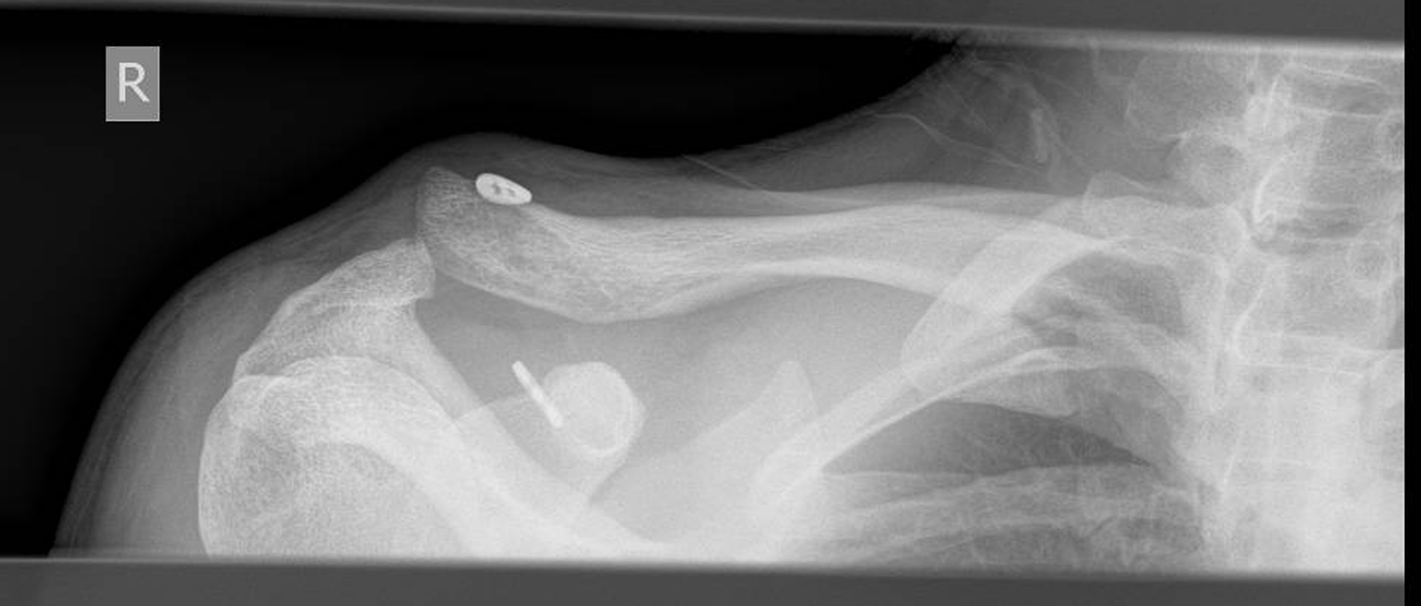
Example of lateral subluxation of the coracoid endobutton leading to loss of radiographic reduction.
